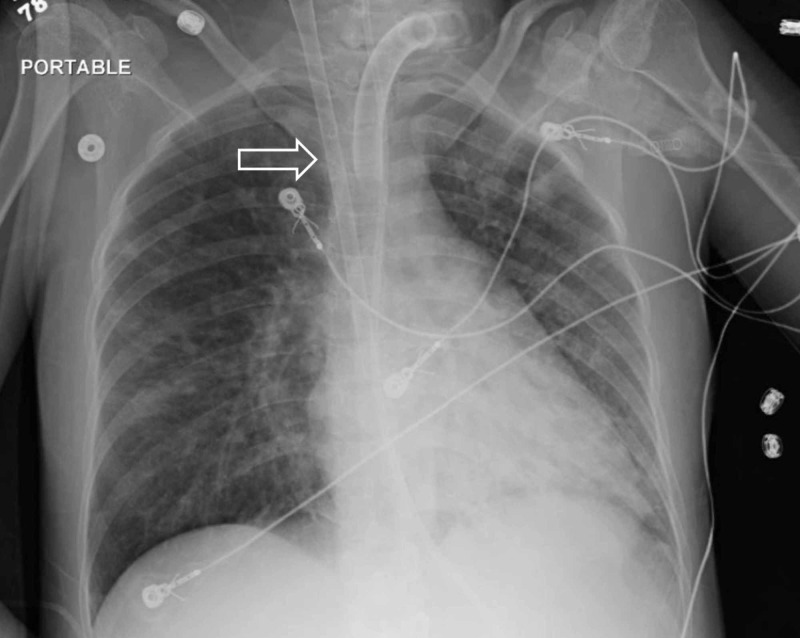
Chest X-ray on hospital day 31, showing resolved ARDS and ECMO cannula in situ (white arrow). ARDS, acute respiratory distress syndrome; ECMO, extracorporeal membrane oxygenation
